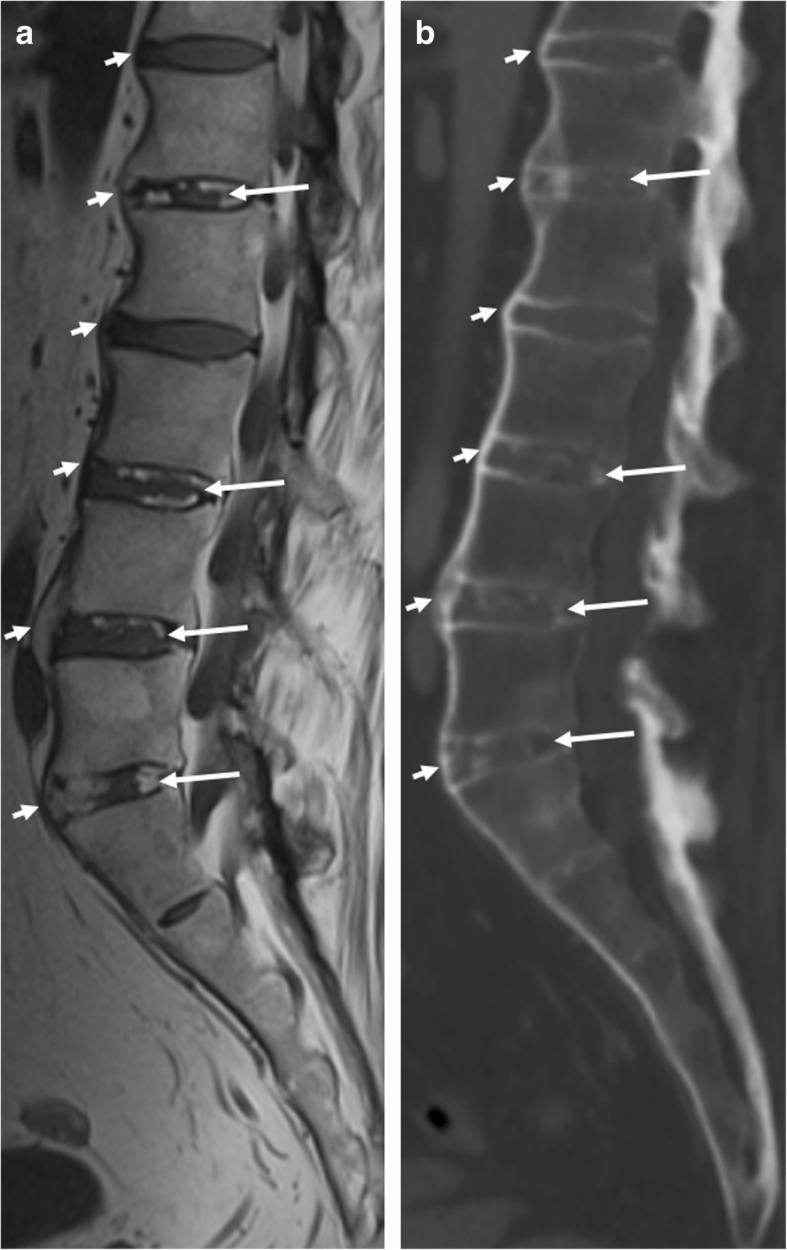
Discal high signal intensity on T1-weighted MR images. a Sagittal T1-weighted MR images of the lumbosacral spine acquired in a 45-year-old man with SpA show signal intensity (long arrows) similar to that of adipose tissue within the intervertebral disc. b Computed tomography (CT) of the same intervertebral discs show discal calcification (long arrows). Also, notice the discordance in the visibility between CT and MRI for the evaluation of anterior bridging syndesmophytes (short arrows)
